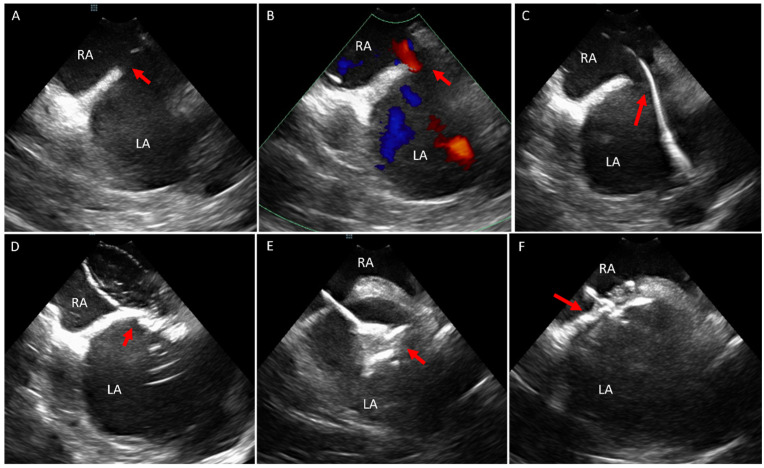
(A,B) ICE catheter positioned in the right atrium looking at the interatrial septum and visualizing the ostium secundum ASD (red arrow) without color Doppler (A) and with color Doppler (B) showing left to right shunt. (C) Wire (red arrow) across the ostium secundum ASD towards the left upper pulmonary vein. (D) Balloon occlusion of the defect (red arrow). (E) Deployment of the left atrial disc of the atrial septal defect occluder device (red arrow). (F) Deployment of the right atrial disc of the atrial septal occlude device (red arrow) and showing a good alignment of the device with the atrial septum. ICE, intracardiac echo; LA, left atrium; RA, right atrium.
